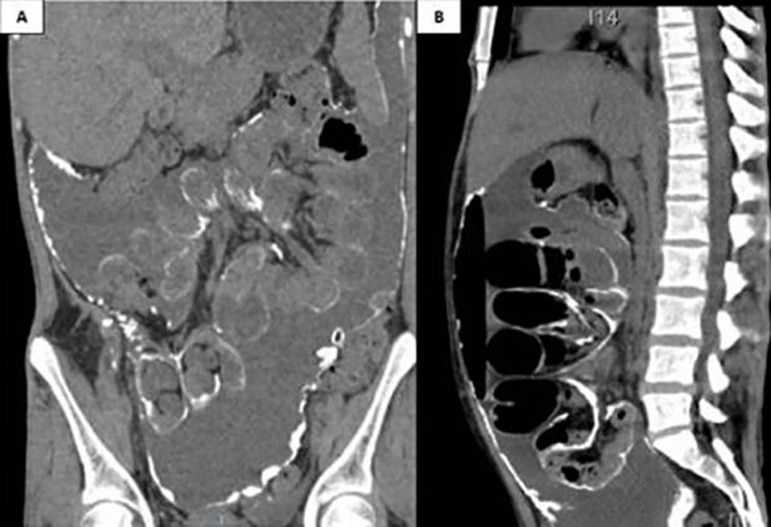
Computed tomography scans of the abdomen and pelvis; coronal view A) and sagittal view (B) showing a thickened and calcified parietal and visceral peritoneum, swollen bowel loops, pneumoperitoneum, ascites, and deposition of material in the peritoneal flexure.
